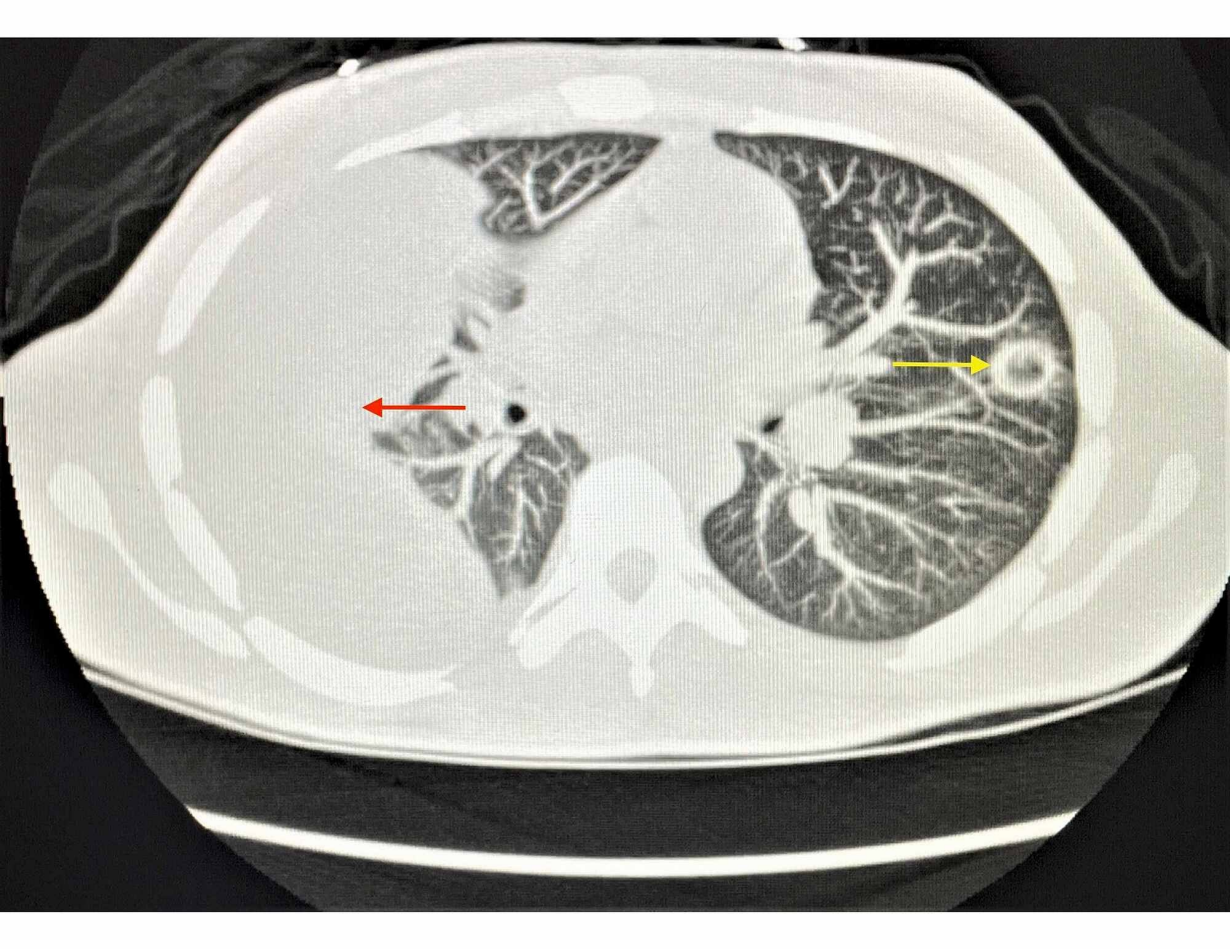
Reformatted CT chest without contrast showing right-sided loculated pleural effusion (red arrow) and left-sided cavitary lung nodule (yellow arrow) prior to pigtail catheter placement. CT, computed tomography
